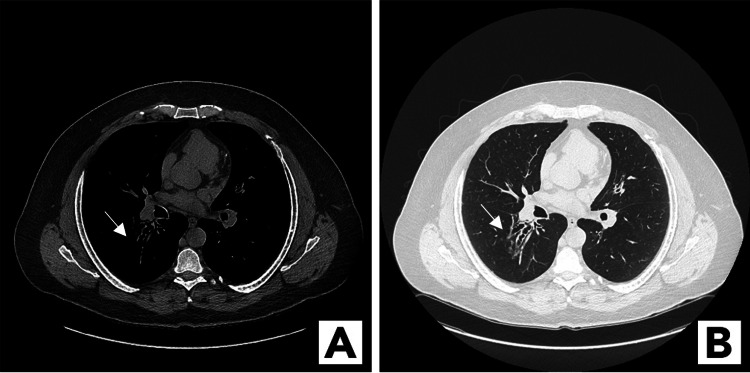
Bronchiectasis Mediastinal window (A) and lung window (B) CT showed hyperdense tubular opacities and dilated bronchi with inspissated mucus, consistent with bronchiectasis (arrows) CT: computed tomography
